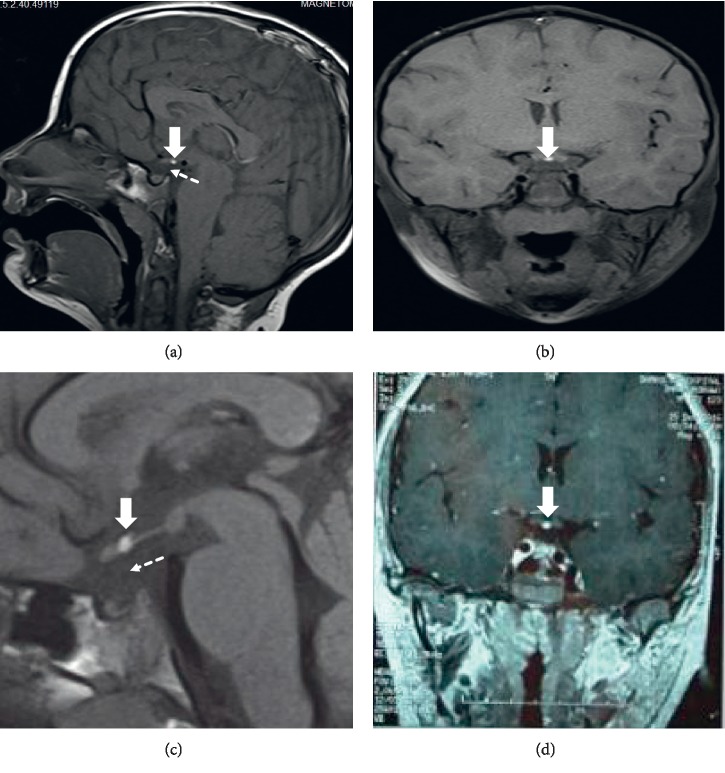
(a) The first patient's sagittal MR image without contrast for the pituitary; the solid arrowhead points to the posterior pituitary lobe (bright spot), while the dashed arrowhead points to the supposed pituitary stalk which is absent. (b) Coronal image without contrast for the first case shows the ectopic bright spot close to the optic chiasm. (c) Noncontrast sagittal view for the pituitary in the second case where the solid arrow points to the ectopic bright spot and the dashed arrowhead points to the absence of pituitary stalk. (d) Contrast coronal image for the second case.
